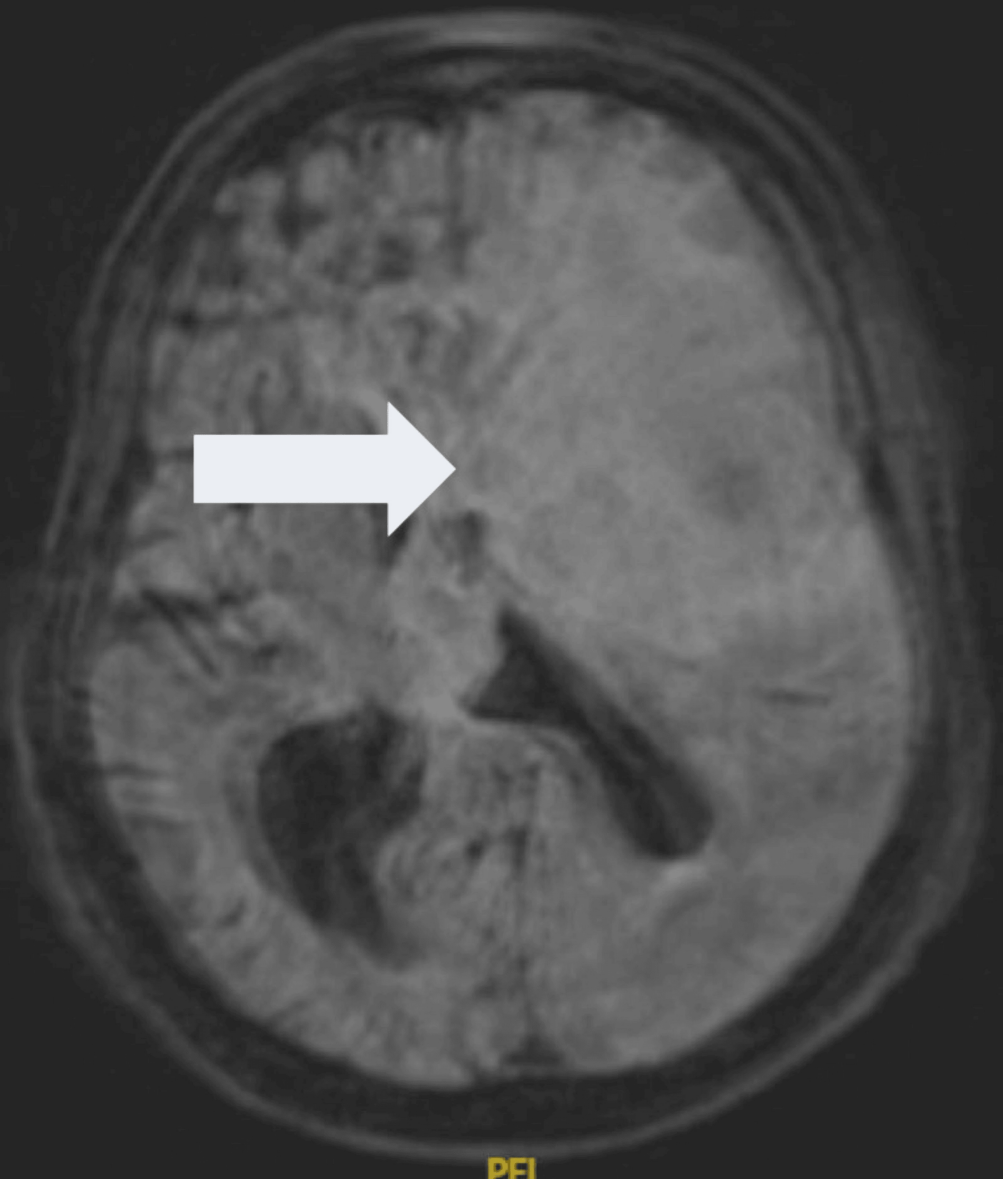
MRI of the brain of patient 1 with RPCNSL There is a large, heterogeneous, centrally necrotic mass in the left frontal lobe and basal ganglia region, with extensive surrounding vasogenic edema and mass effect causing effacement of the sulci. The mass extends into the lateral ventricles and involves the body and genu of the corpus callosum. RPCNSL = recurrent central nervous system lymphoma
